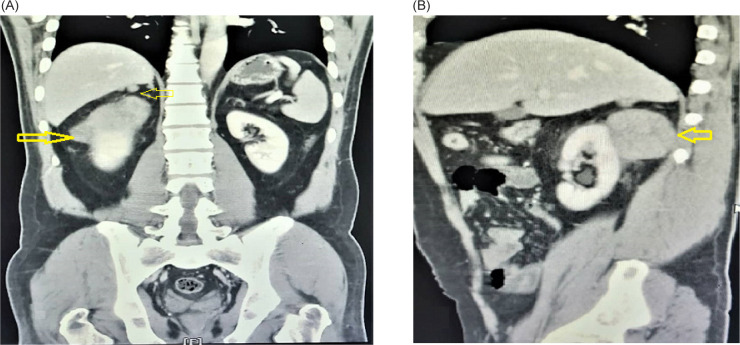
A computerized tomography (CT) scan showed: (A) Mass in the right kidney (arrow), two masses were attached to the liver tightly (arrow). (B) Oblique view of mass in the right kidney with low enhancement (arrow).
